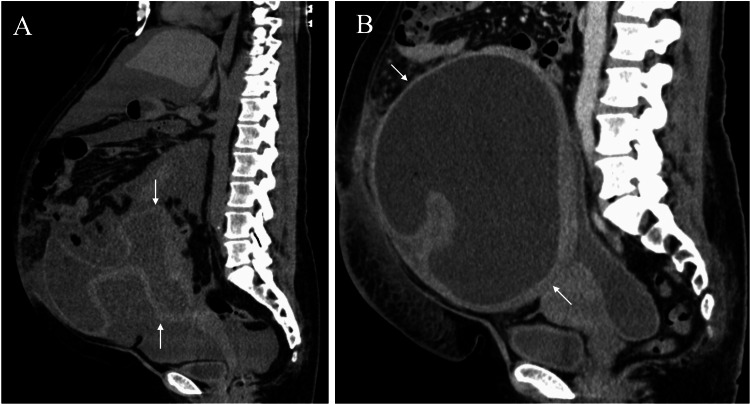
Computed tomography (CT) findings A. Non-contrast sagittal abdominal CT two weeks after the initial consultation showed massive ascites, membranous structures of unknown origin (arrows), and no evident masses. B. Non-contrast sagittal abdominal CT six weeks after the initial consultation showed a large cystic lesion (arrows).
